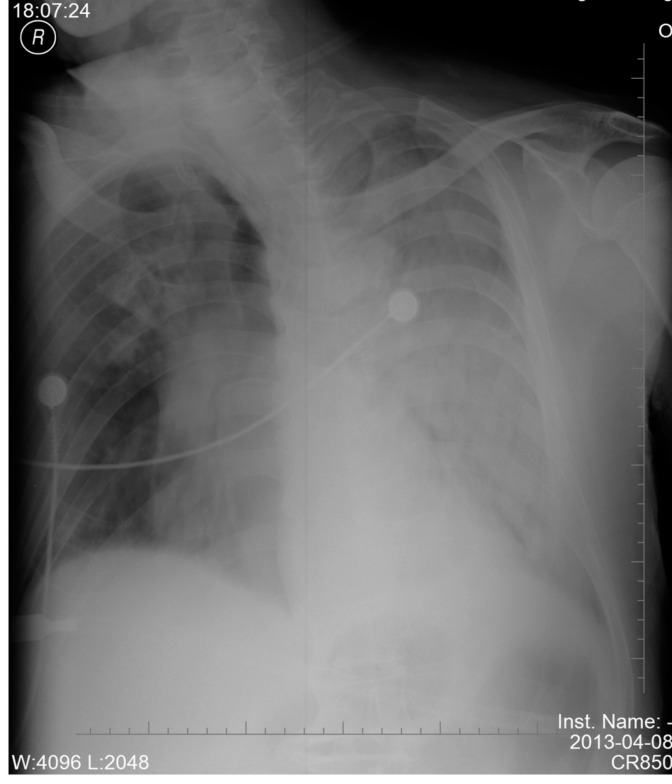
On April 8^th^ 2013, the chest radiograph showed the rapid progression of the lung lesions, with two pulmonary diffuse ground glass opacities.
